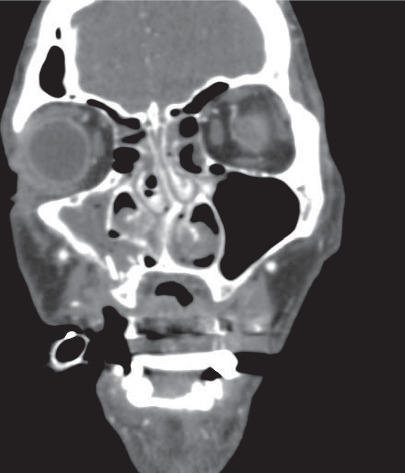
Preoperative coronal computed tomography showing a right anterior inferior maxillary sinus fracture with a hematoma in the right maxillary sinus.
